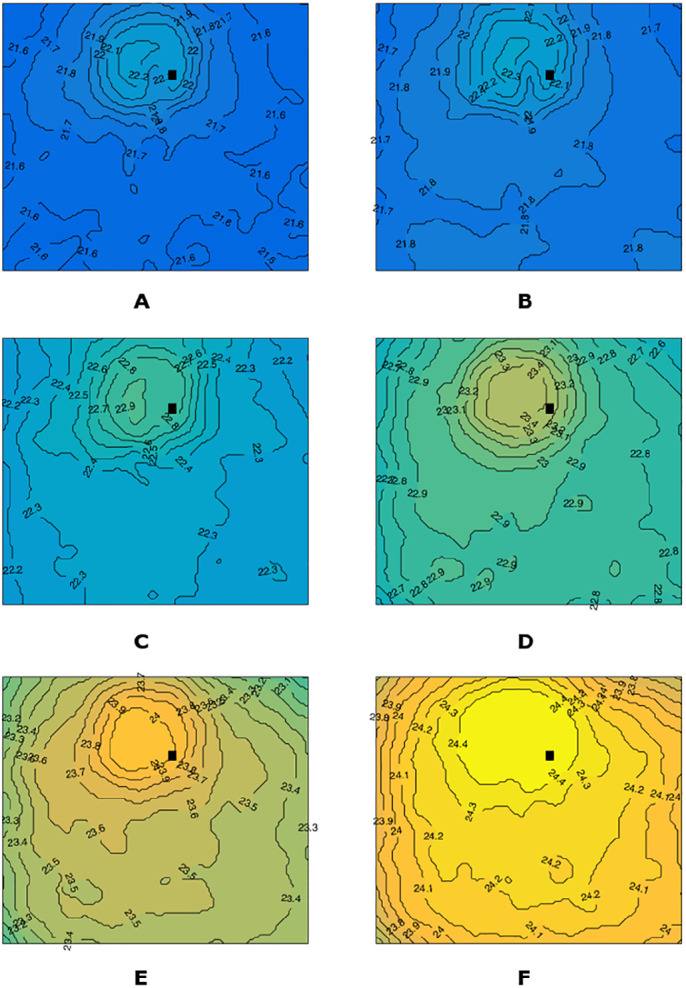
Temperature maps at different times *t* for the ultrasound VIT-L probe: (A) *t* = 0; (B) *t* = 1 minutes; (C) *t* = 2 minutes; (D) *t* = 3 minutes, (E) *t* = 4 minutes, (F) *t* = 5 minutes. Stroke set at 60 µm. The area depicted in the picture has a side of approximately 2 cm. The black square in the images indicates the approximate position of the probe tip. Temperature ranged from 21°C to 24.5°C.
